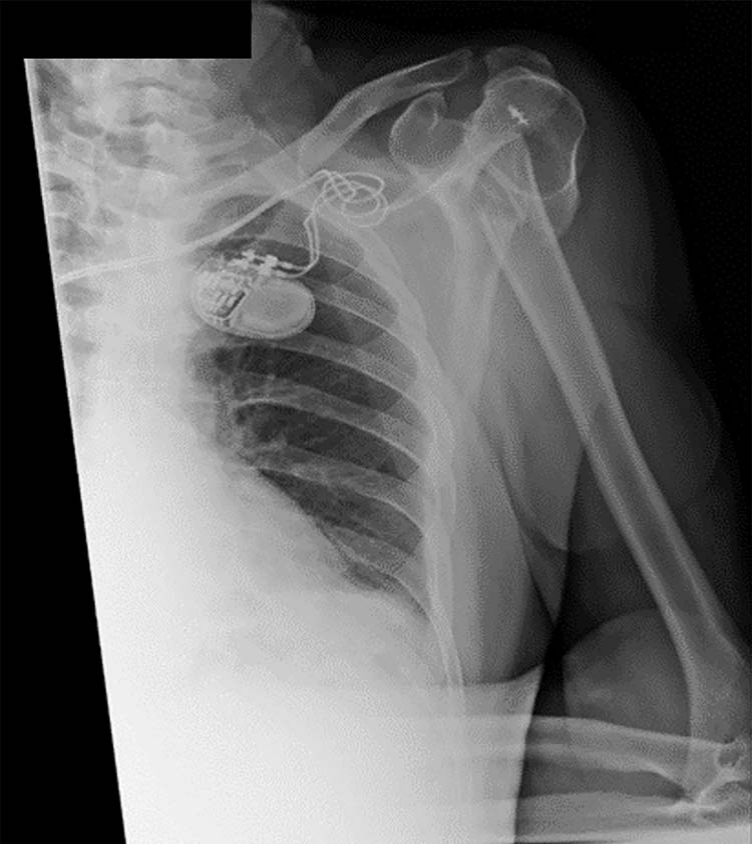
Antero-posterior view X-ray taken on day of admission, showing a left surgical neck of humerus fracture with anterior displacement of the proximal humeral shaft.
